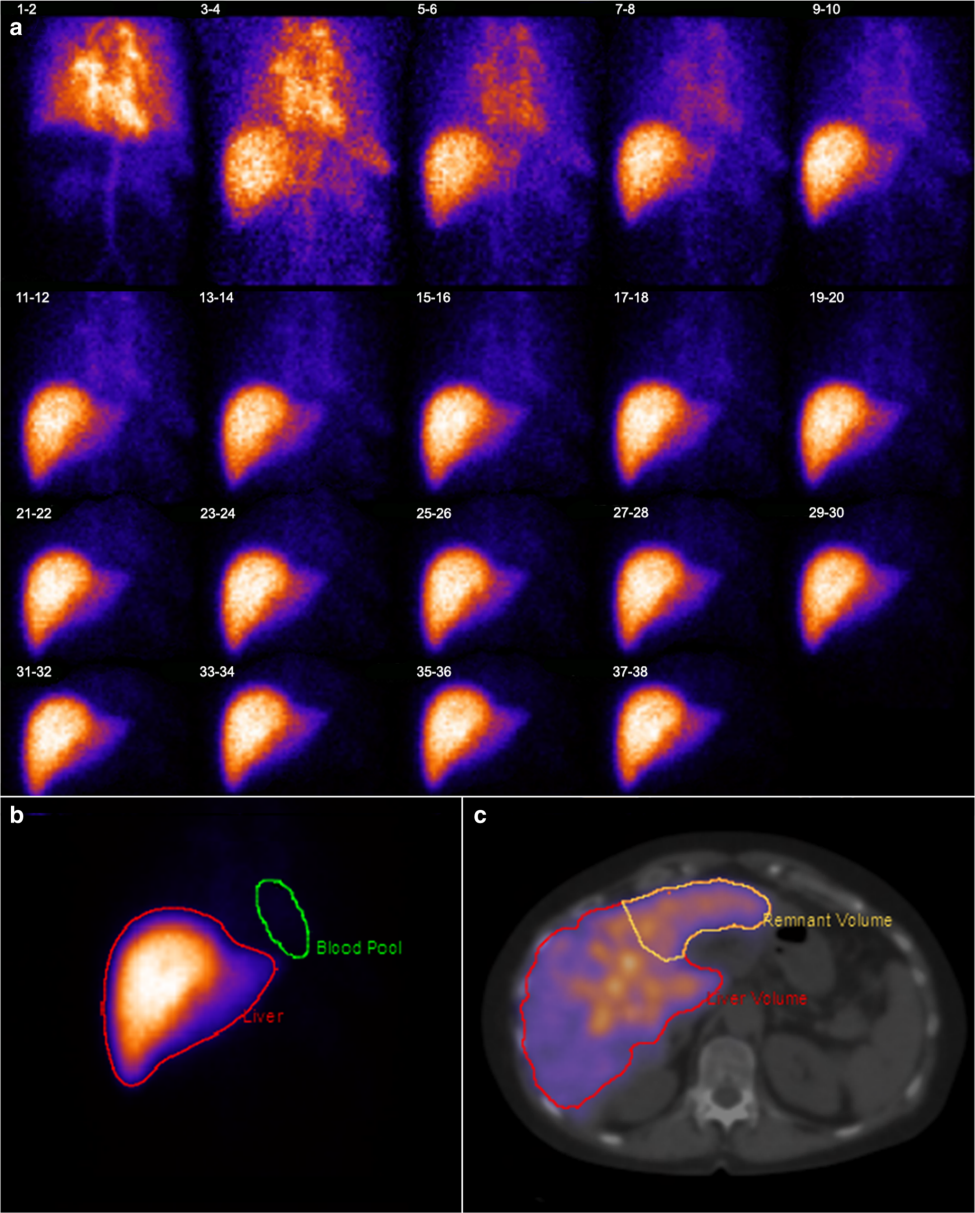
Hepatobiliary scintigraphy with series scintigram (a), ROI on summed images (b), and FRL delineation on SPECT/CT (c)
